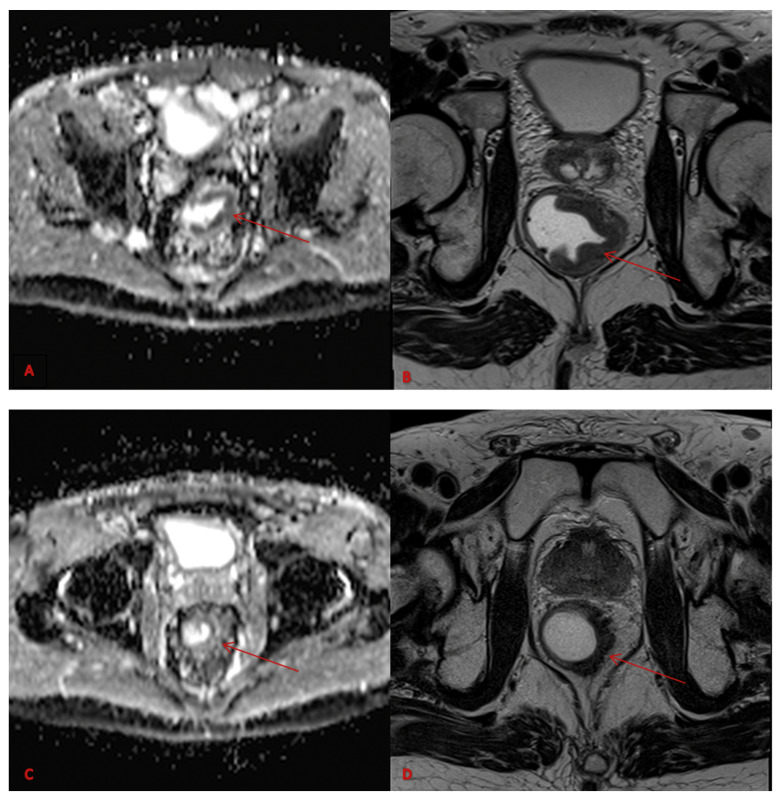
ADC map pre-therapy (A), Axial MRI T2w pre-therapy (B), ADC map post-therapy (C) and Axial MRI T2w post-therapy (D). Images refer to rectal cancer (red arrows).
